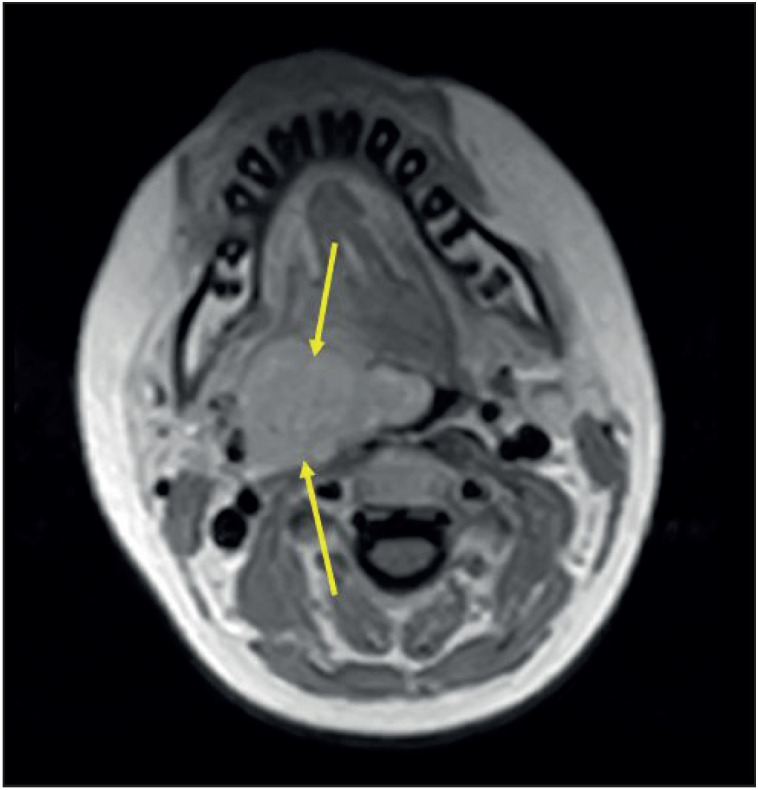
T1-weighted post contrast image shows diffuse homogeneous enhancement of the mass lesion (arrows).
